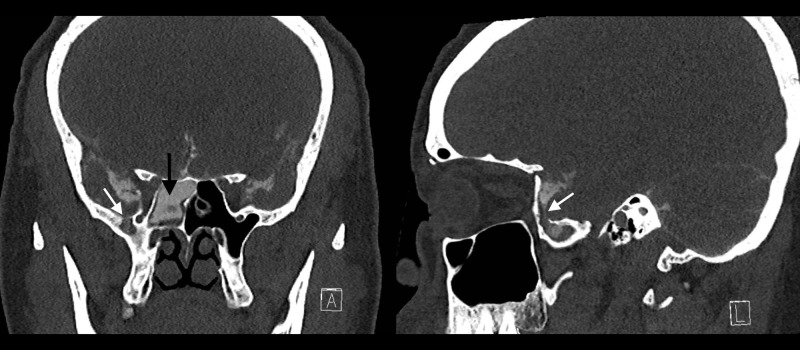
CT cisternogram post-contrast, coronal and sagital view Computed tomography (CT) shows that the contrast material within the middle cranial fossa appears to extend through this defect into the lateral aspect of the right sphenoid sinus.
